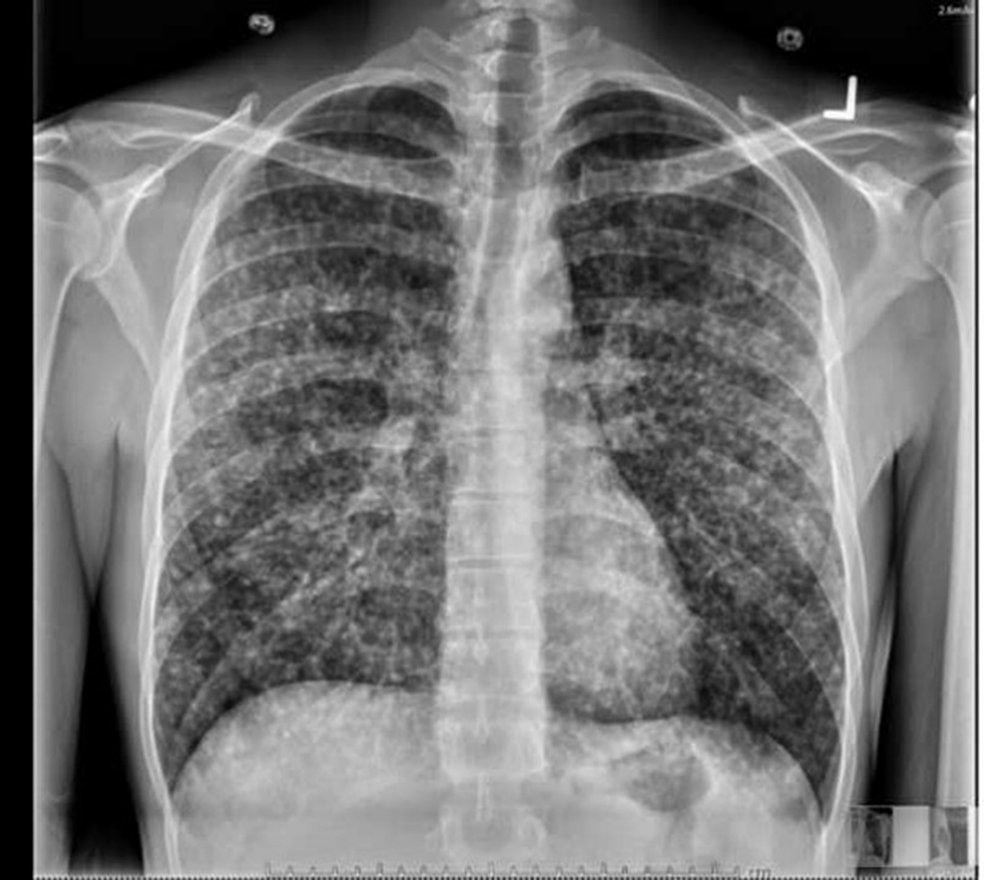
Posterior-anterior chest x-ray on admission
